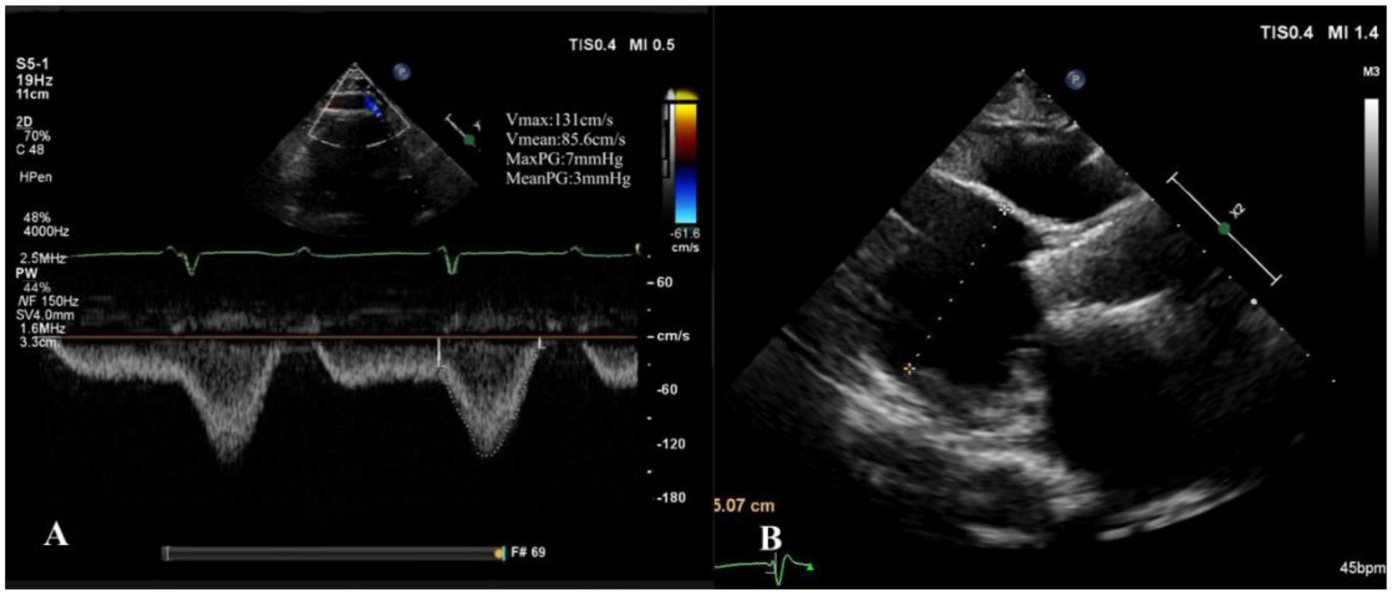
One-month postoperative echocardiographic follow-up. (A) Spectral Doppler showing no evidence of aortic regurgitation; (B) long-axis view demonstrating no late prosthetic valve migration.
